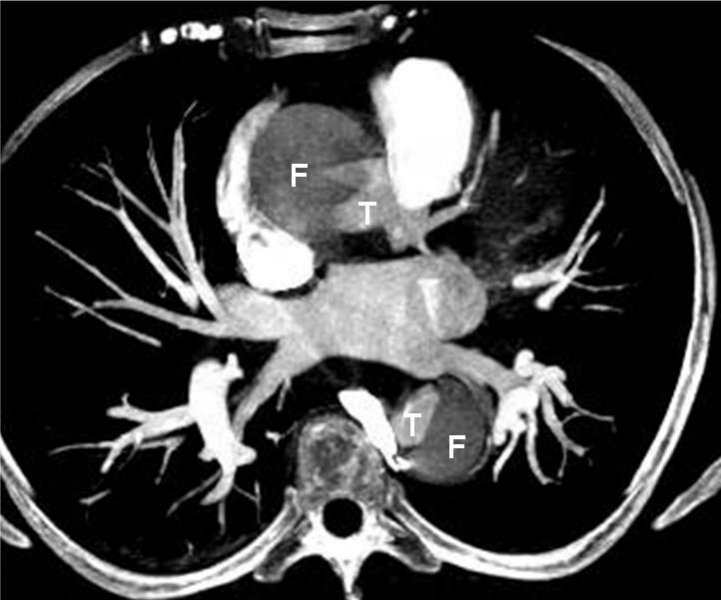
Multidetector Computed Tomography Angiography (MDCTA): Axial plane demonstrates an intimal flap that separates the false (F) from the true lumen (T) in the ascending and descending aorta, diagnostic of a Stanford type A dissection.
